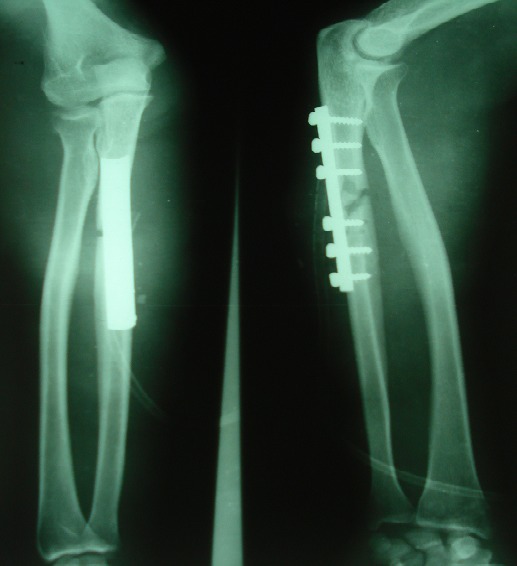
Radiography post operative control of the second patient
